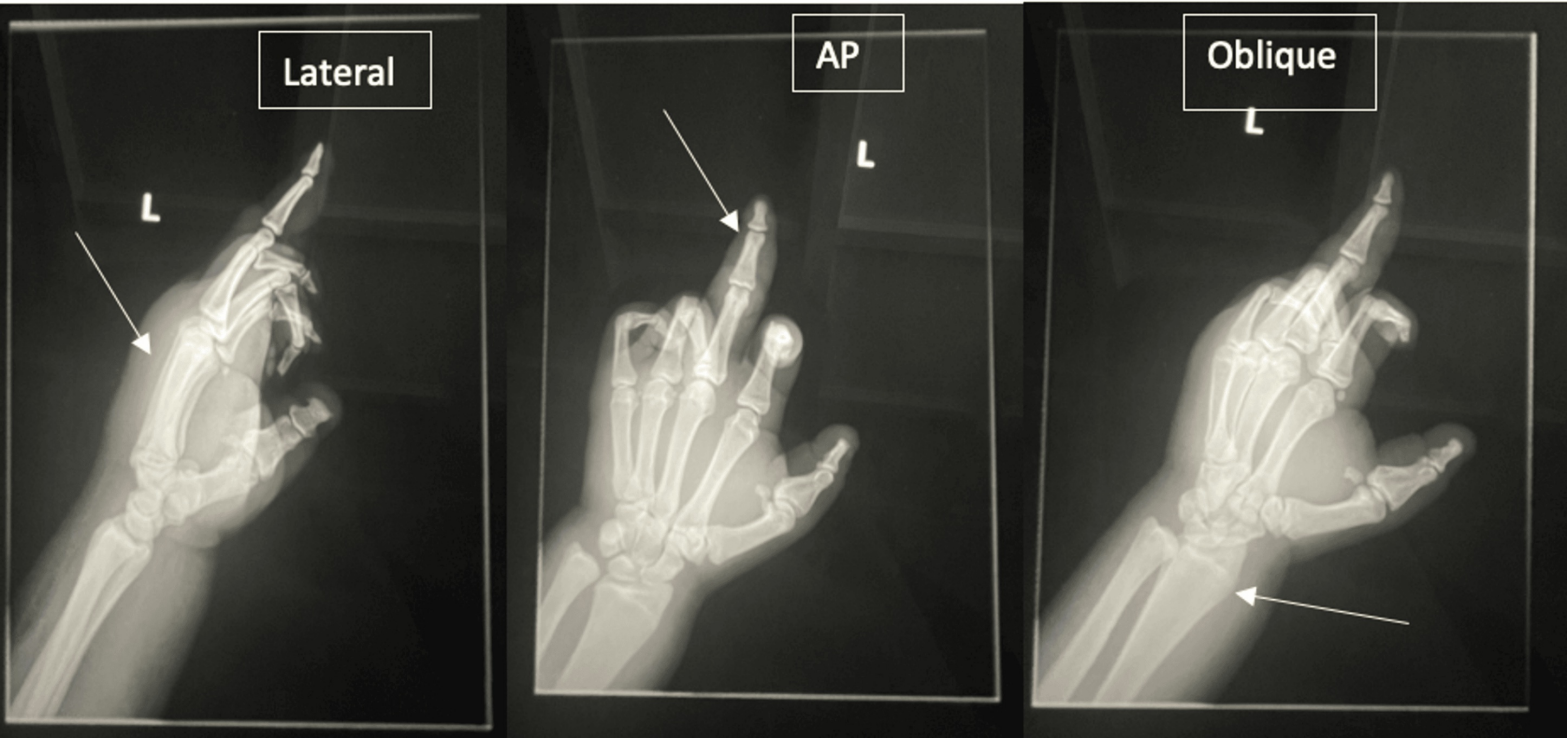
Lateral, AP, and oblique X-ray images of the patient’s left hand. No fracture or malalignment is present. Significant soft tissue swelling of the distal forearm, hand, and third digit is indicated by the arrows.
